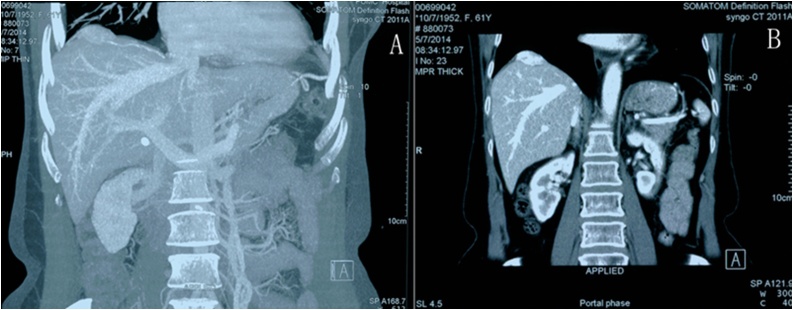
Computed tomography shows an arched lesion on the top of the liver.
